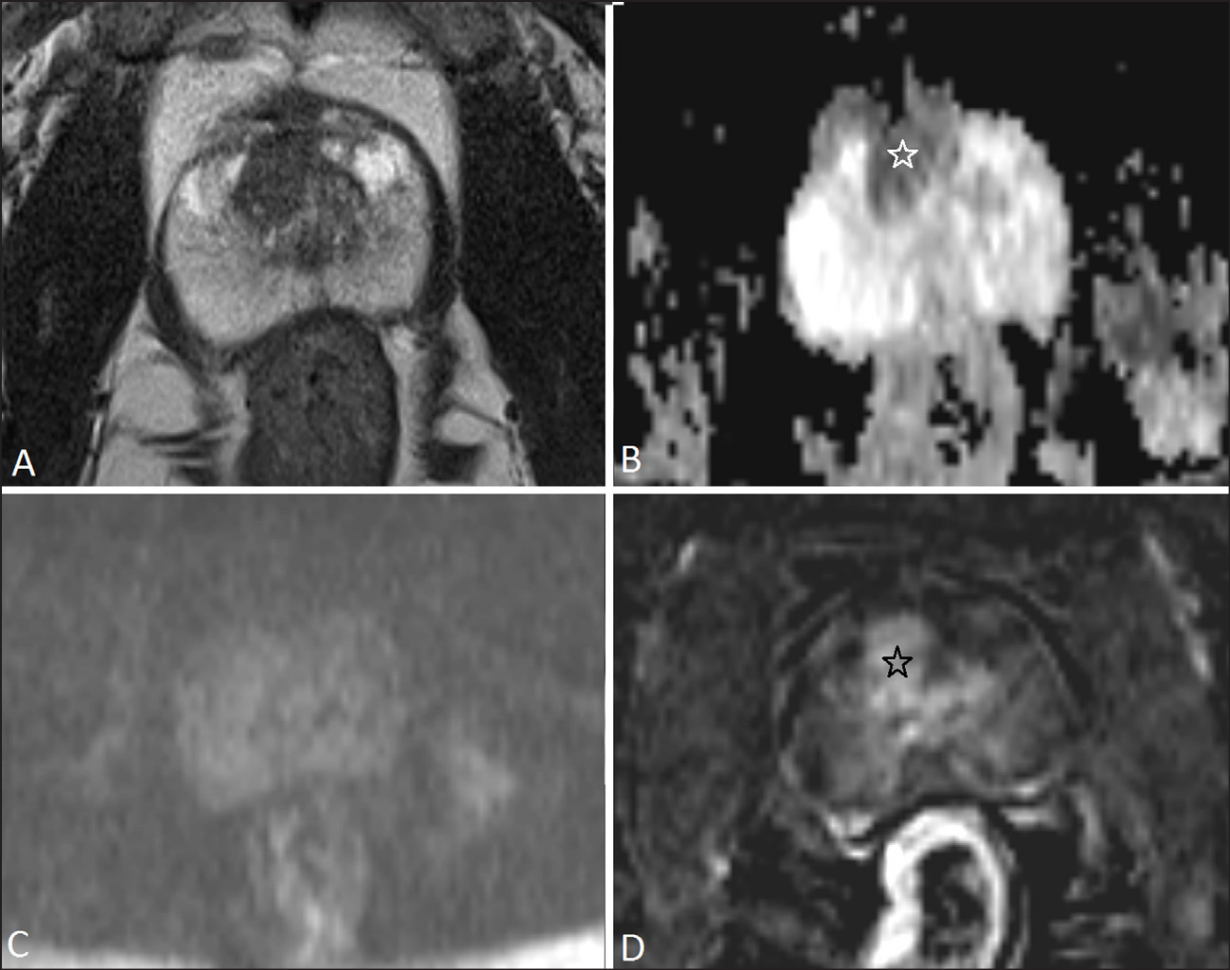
A 56-year-old man with PSA of 11.2μg/L. On T2-WI (a) the PZ shows a homogenous high signal intensity (PI-RADSv1 score 1; PI-RADSv2 score 1) and in the TZ a well-margined homogenous hypointense area is noted (PI-RADSv1 score 2; PI-RADSv2 score 2). On DWI this area in the TZ shows low ADC value (b) (white star) and is isointense on high-b-value image (c) (PI-RADSv1 score 4; PI-RADSv2 score 4). On DCE this area shows strong contrast enhancement (black star) (PI-RADSv1 score 4; PI-RADSv2 positive). With the PI-RADSv1 scoring system this patient was assigned an overall assessment score 4, based on a subjective overall impression of the findings in all modalities. With the PI-RADSv2 scoring system, T2-WI in the TZ was scored 2, and since T2-WI is the dominant modality in the TZ the overall assessment score was also 2. Prostate biopsy followed by radical prostatectomy in this patient showed a Gleason 3+4 prostate cancer in the TZ.
